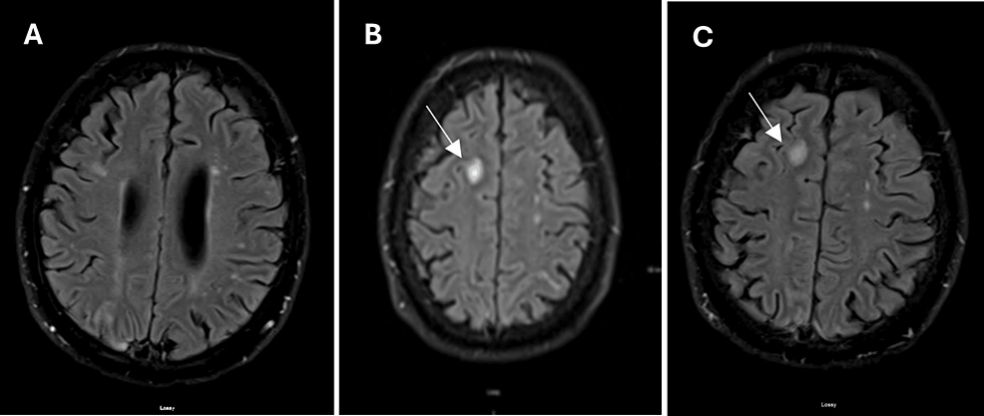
MRI with intravenous contrast showed multiple areas of abnormal enhancement within the brain parenchyma, including a ring-enhancing lesion in the right frontal lobe MRI: magnetic resonance imaging
